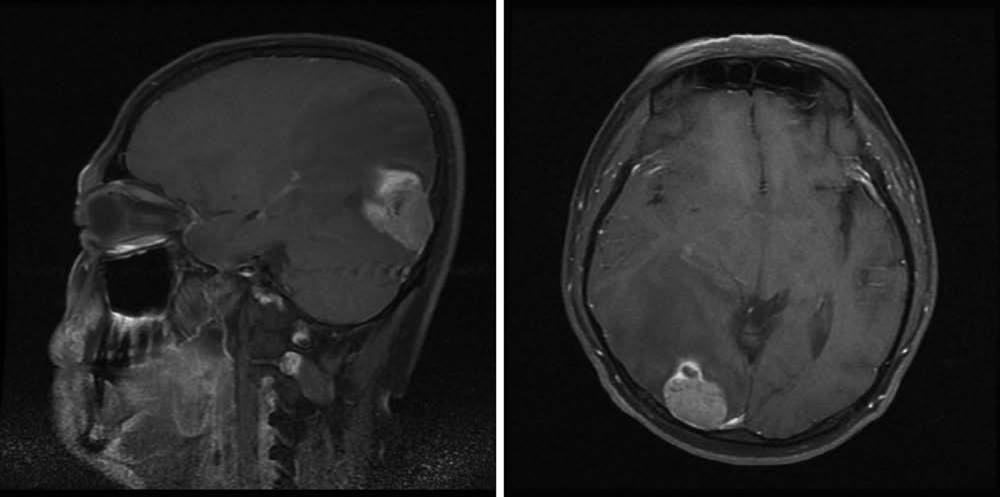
Brain magnetic resonance imaging demonstrates a focal lesion at the right occipital lobe with compression of the posterior horn and of the right lateral ventricle.
